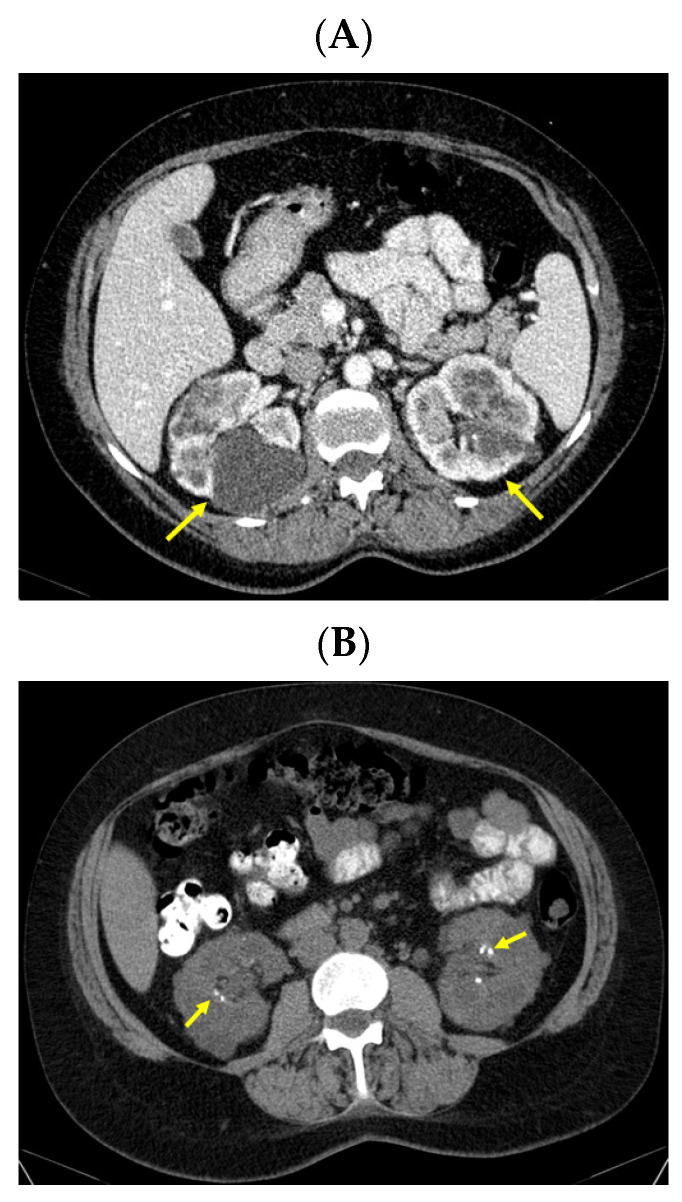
Intravenous contrast abdominal CT showing: (A) both kidneys with multiple cystic lesions both located in and protruding from the renal cortex bilaterally, the posterior part of the right kidney with a cyst of 6.27 by 5.36 by 6.01 cm with mass effect on the phyllo-caliceal system and protruding from the kidney (the yellow arrows show cystic kidney disease) and (B) bilateral renal microstones in primary hyperparathyroidism in yellow (axial plane).
